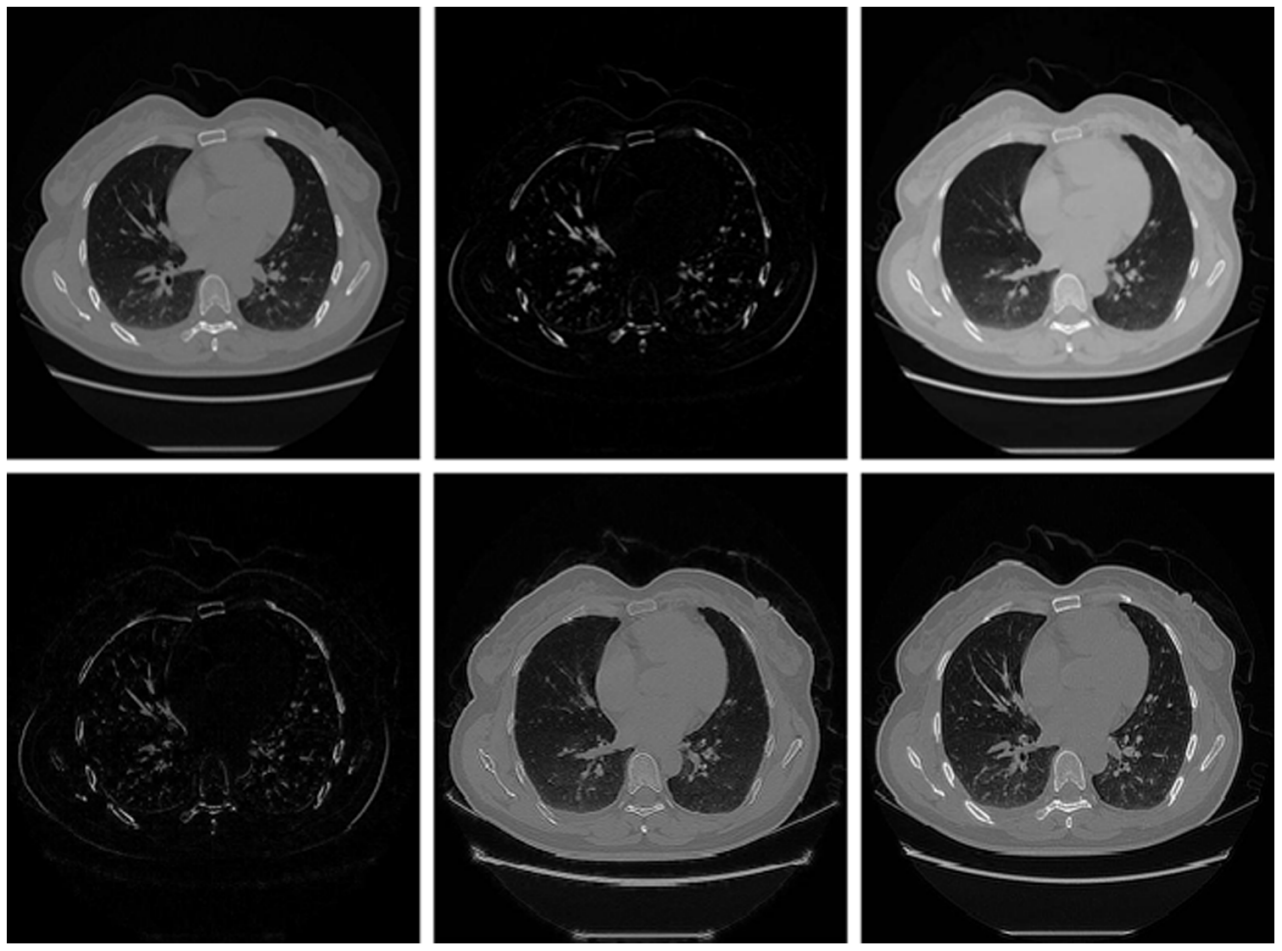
Shows restoration procedure of sparse and low-rank decomposition. From left to right, from top to bottom: the first image of the chest CT image sequence, the sparse component, the low-rank component, the recovery image of the sparse component, the recovery image of the low-rank component and the recovery CT image. We can see that the detailed information and the visual effect of the CT image after restoration are obviously improved.
